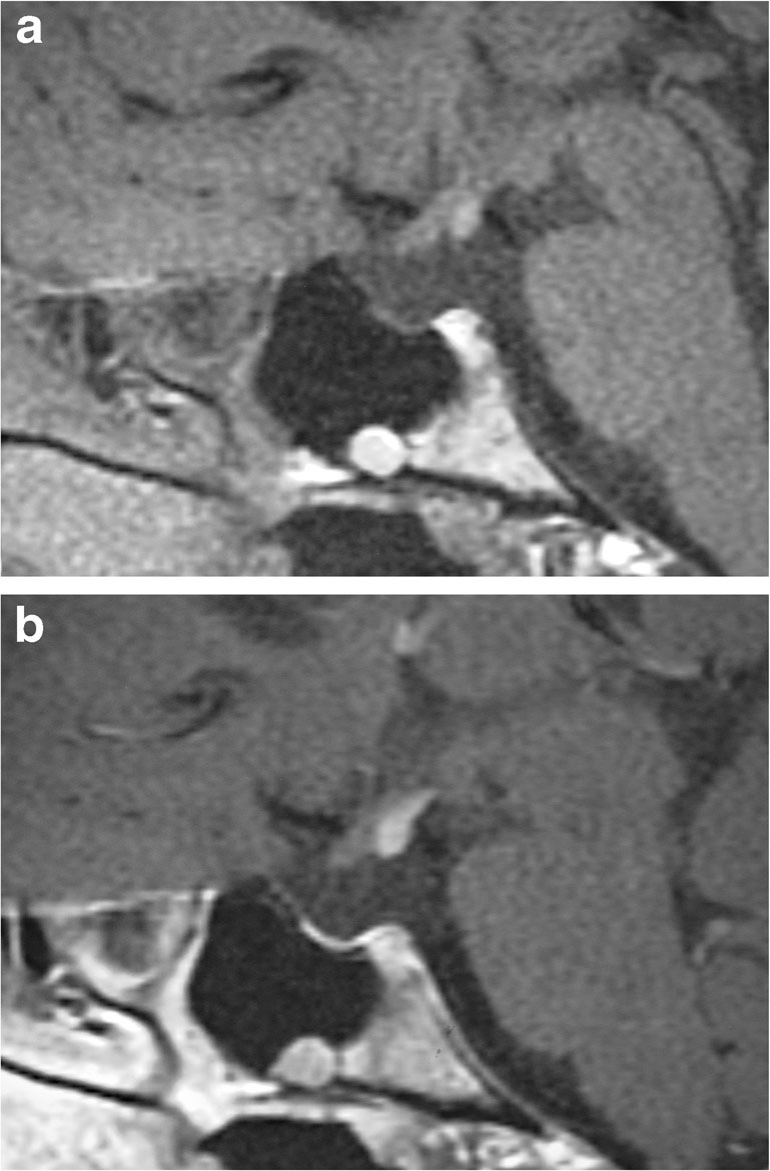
Severe hypoplasia of the anterior pituitary gland. Pre-contrast (a) and post-contrast (b) MR images show severe hypoplasia of the anterior pituitary gland which shows only minimal contrast enhancement along the floor of the sella. The ectopic posterior pituitary is located adjacent to the optic chiasm
